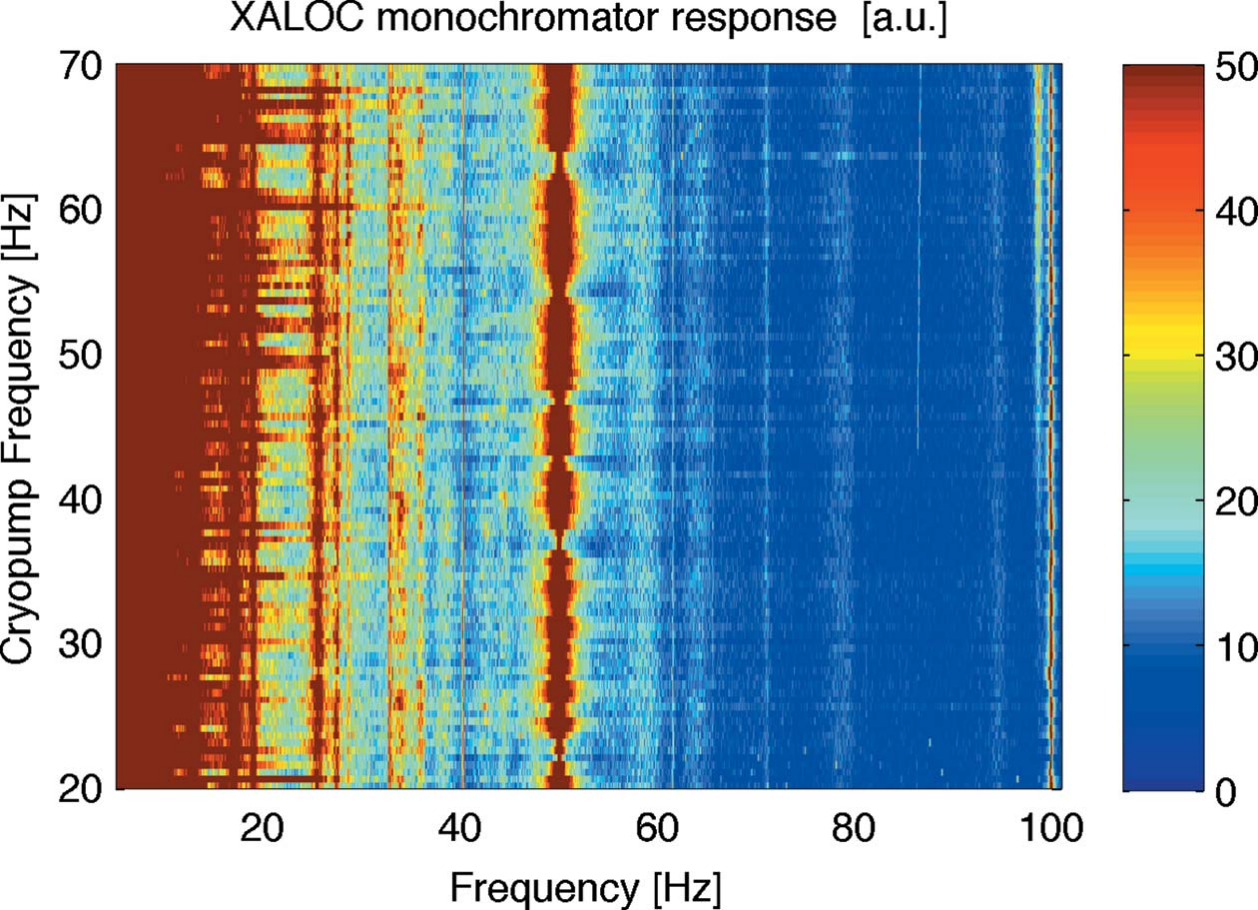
Vibrational behaviour of the monochromator as measured by laser interferometry, with the laser beam following the X-ray beam path, at a cryopump frequency that varied from 20 to 70 Hz (in 1 Hz steps). The excited frequencies (*e.g.* 19, 25, 50 Hz) are independent of the cryopump regime, and are due to resonances of the metrology set-up and the electronic noise.
